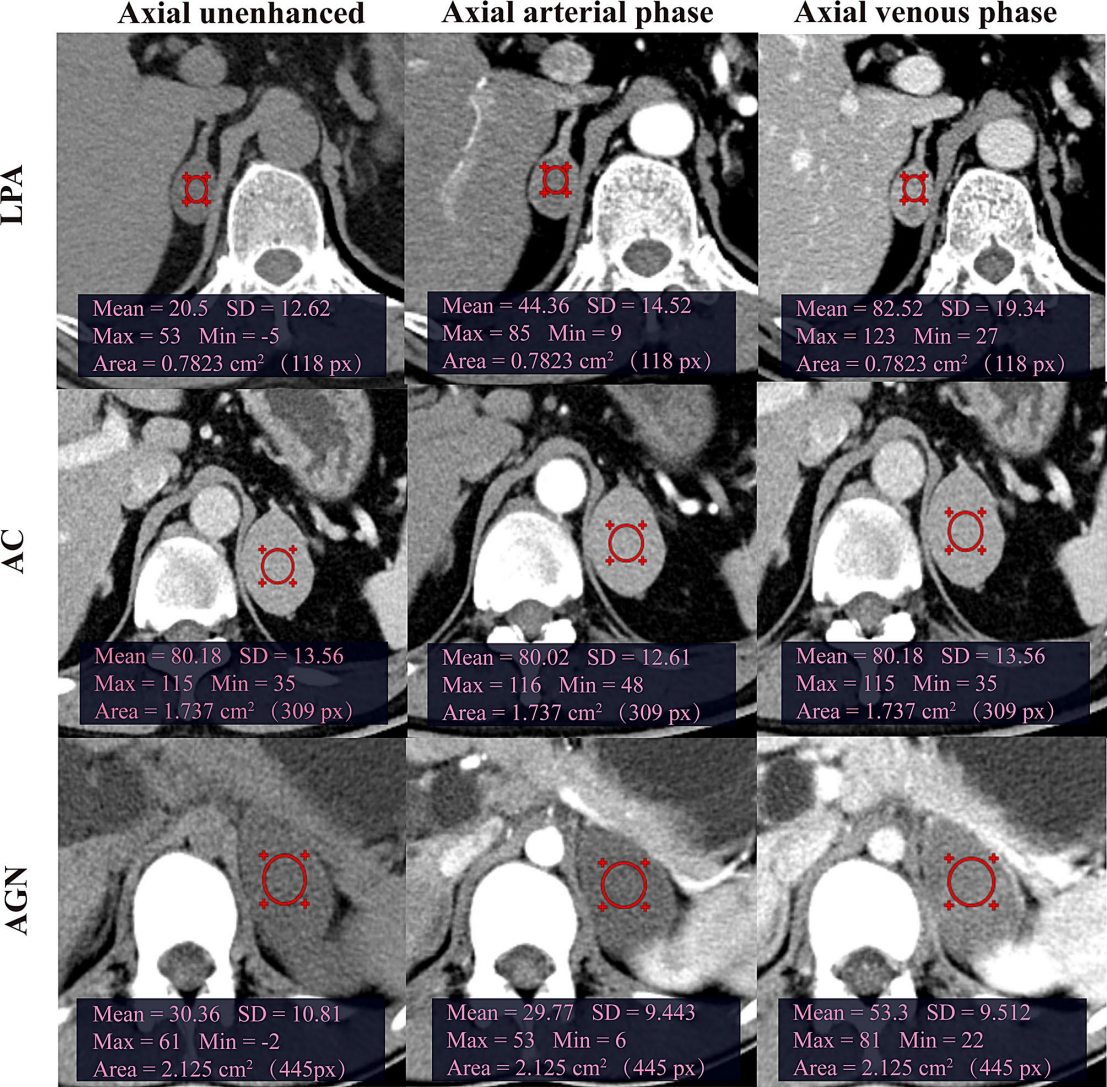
(1) The patient with a right LPA, and the CTu, CTa, and CTv values were 20.50 HU, 44.36 HU, and 82.52 HU, respectively. (2) The left AC patient with CTu, CTa, and CTv values of 80.18 HU, 80.02 HU, and 80.18 HU, respectively. (3) The patient with left AGN, and the CTu, CTa, and CTv values were 30.36 HU, 29.77 HU, and 53.30 HU, respectively. CTu = CT attenuation value of unenhanced phase. CTa = CT attenuation value of arterial phase. CTv = CT attenuation value of venous phase. AC = adrenal cyst, LPAs = lipid-poor adenoma, AGN = adrenal ganglioneuroma
